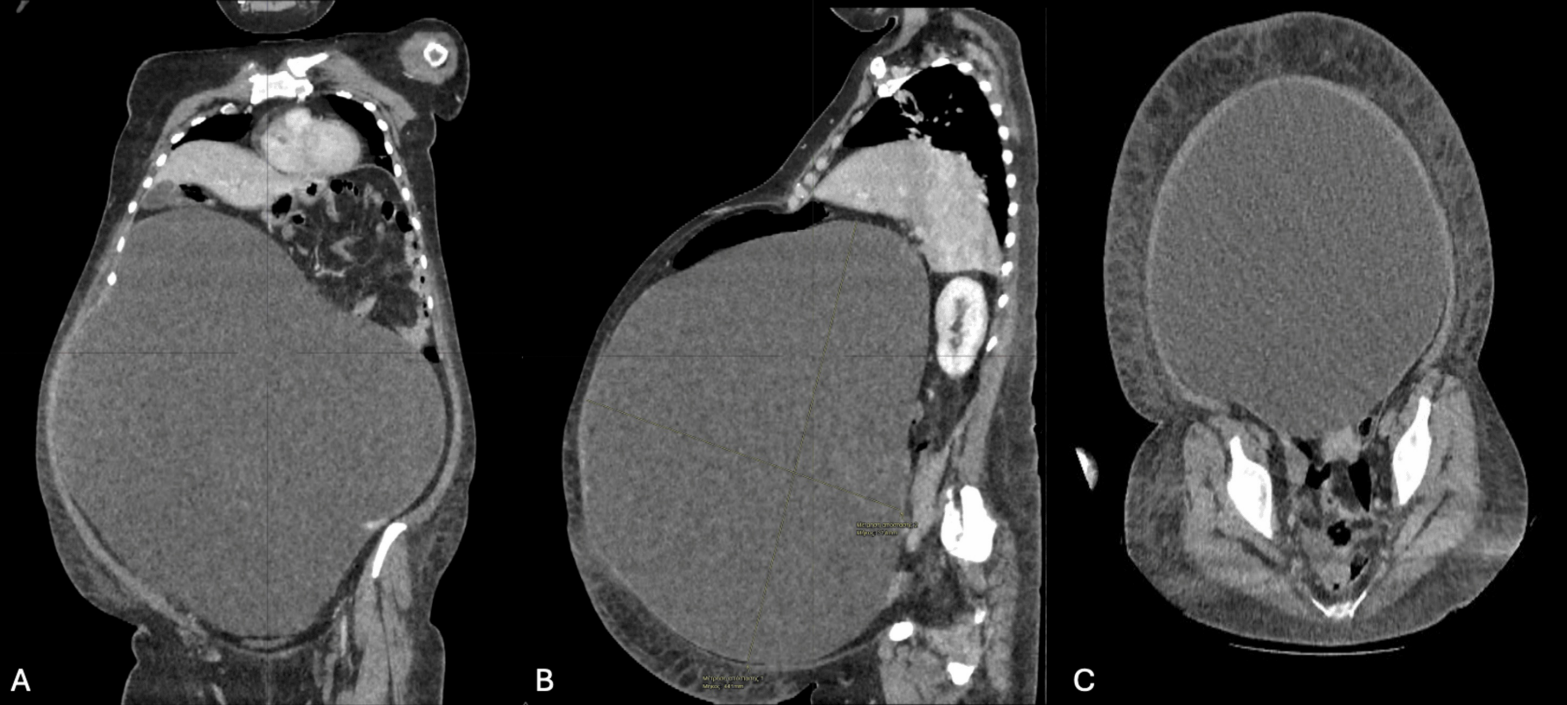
(A) Coronal CT: giant unilocular, low-attenuation cyst occupying most of the abdominal cavity and displacing bowel loops. (B) Sagittal CT: orthogonal calipers demonstrate the cyst’s maximal craniocaudal (44.1 mm) and anteroposterior (31.4 mm) dimensions. (C) Axial CT: homogeneous, thin-walled cystic lesion exerting mass effect on the pelvic organs and great vessels.
